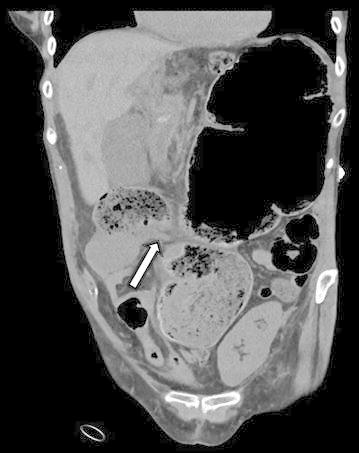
Abdominal/pelvis CT demonstrating significant dilatation of the cecum and proximal allograft ureter (*arrow*)
